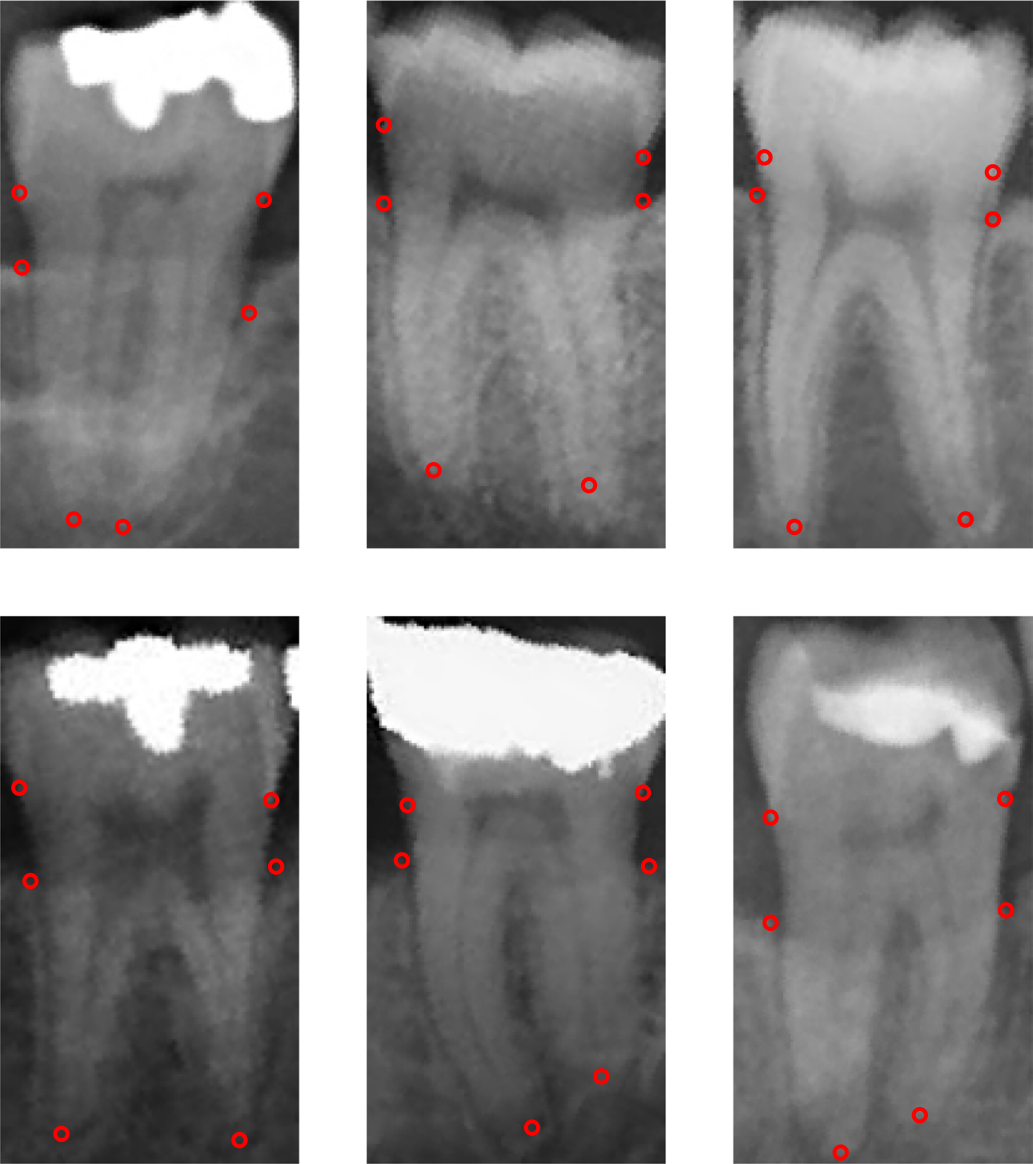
Segmented molar images with PBL points. Legend: “Sample of the resulting segmented molar images (1970) after data processing of labelled panoramic radiographs. Each molar image contains the necessary radiographic points for PBL calculation”
